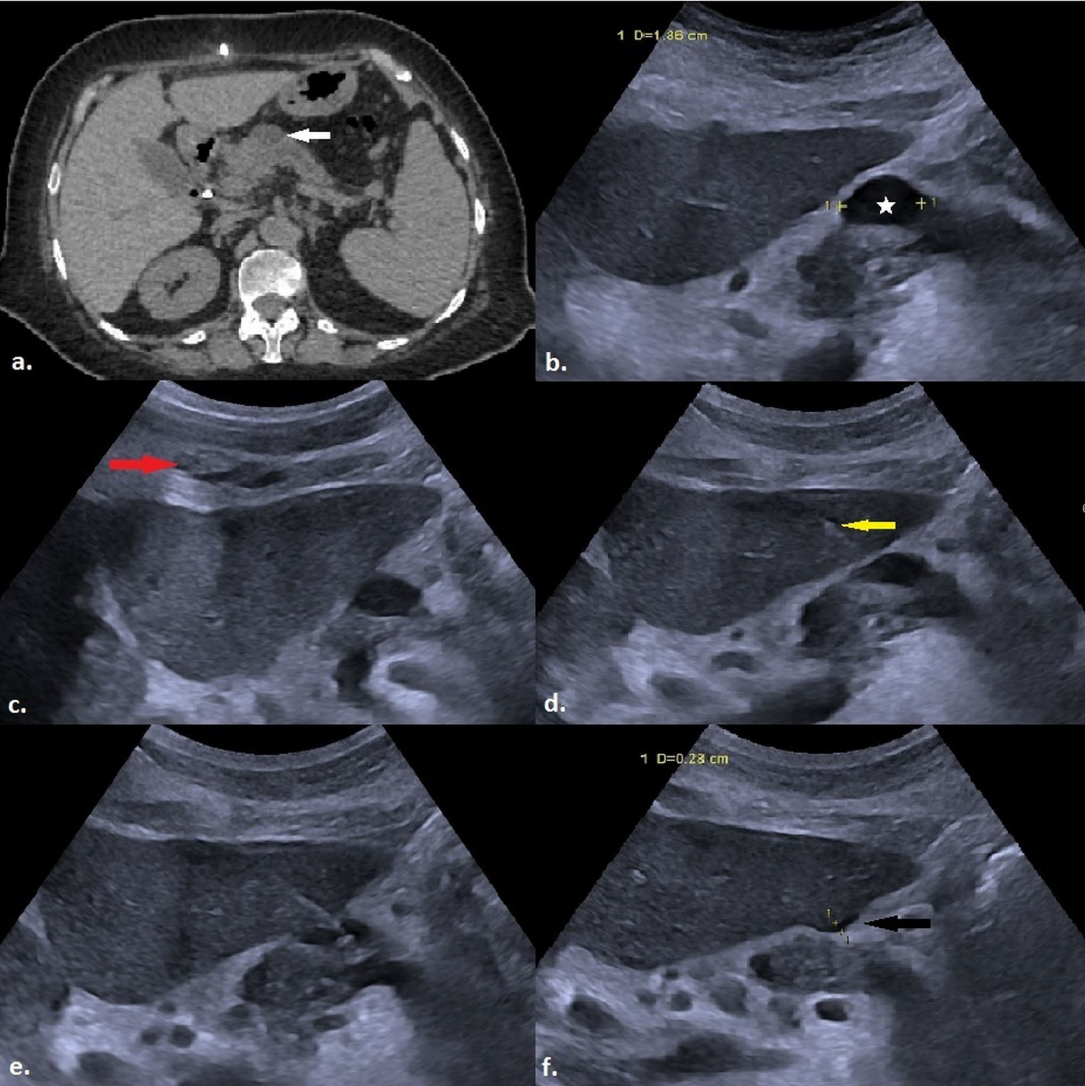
a) A well-defined hypodense lesion (white arrow) is observed in the axial section of a non-contrast abdominal CT scan, located at the neck-body junction of the pancreas. b) The maximum diameter of the same lesion (white star) is measured as 1.86 cm on US imaging. c) Prior to the biopsy planned under US guidance using the CTHa, local anesthesia is administered with approximately 10–20 ml of 2% prilocaine hydrochloride around the anterior liver capsule and subcutaneous tissue (red arrow). d) The 17-gauge introducer (yellow arrow) is advanced completely through the liver parenchyma to reach the lesion. e) US image recorded immediately after the biopsy procedure. f) Post-procedural control US examination reveals a minimal hemorrhage, measuring 0.23 cm in thickness, in the perihepatic area at the procedure site (black arrow). The patient, who showed no abnormalities during follow-up, was discharged without major complications. The pathological examination of the lesion was reported as granulomatous lymphadenitis (CTHa: Complete Transhepatic Approach, US: Ultrasound; CT: Computed Tomography)
